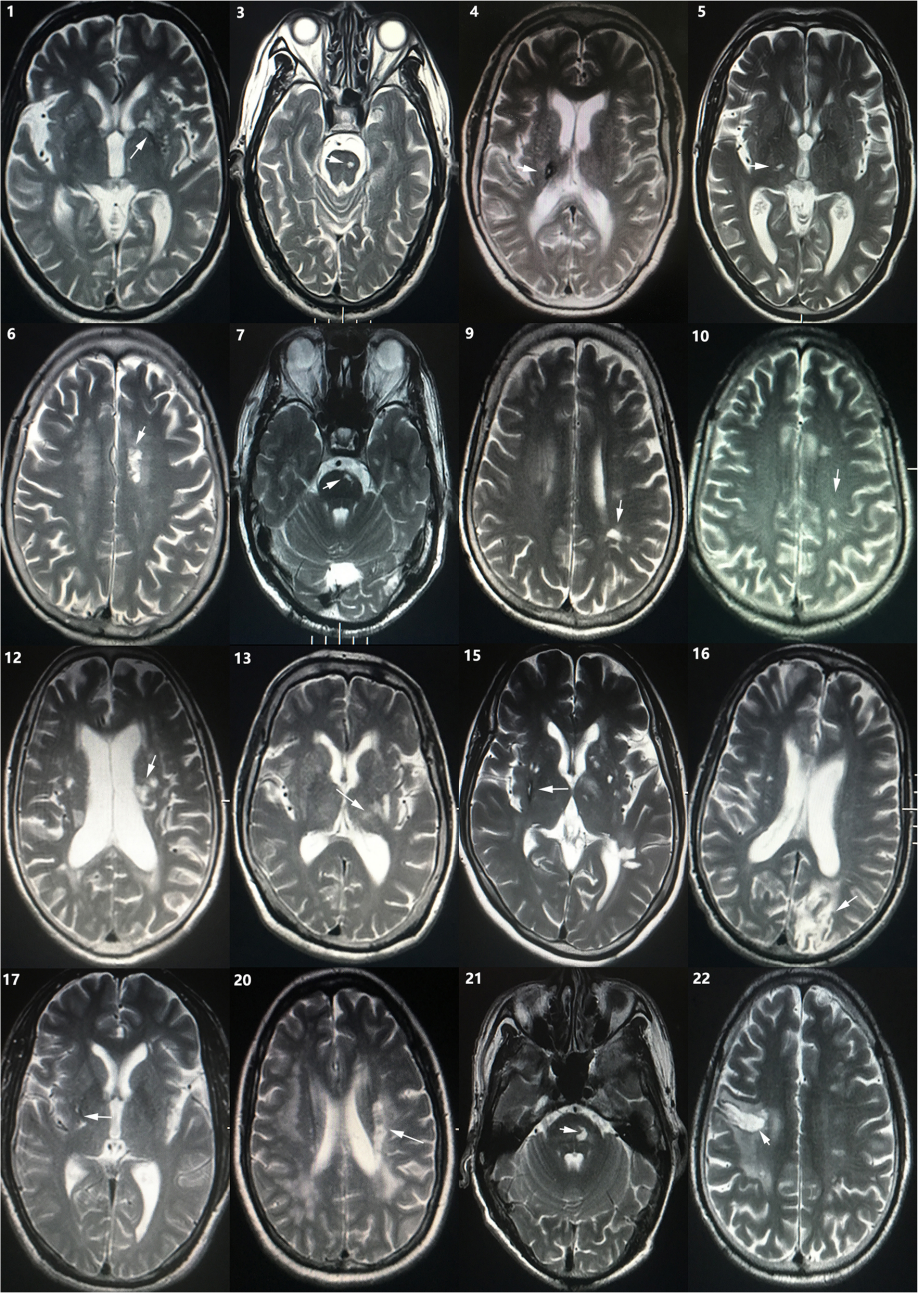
MRI radiographs of patients show responsible lesions of past stroke on T2-weighted images
